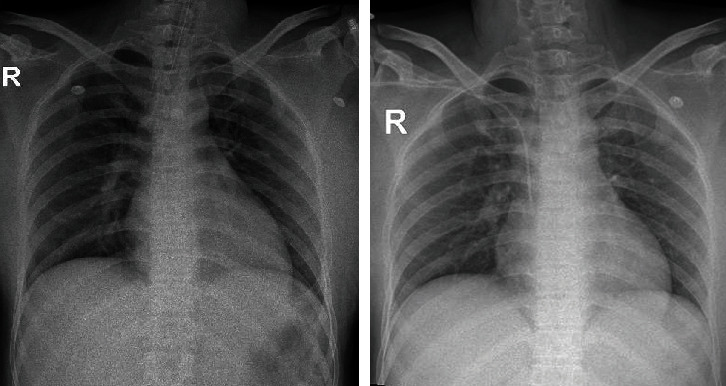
Chest X-ray images on admission (left) and postextubation (right) showed bilateral ground glass opacities consistent with mild COVID-19.
